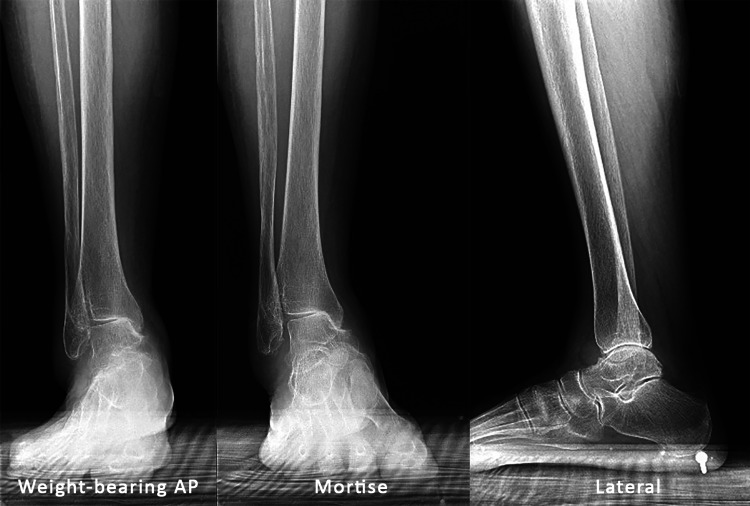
Right ankle: AP, mortise, and lateral radiographs AP, mortise, and lateral radiographs of the right ankle show tibiotalar osteoarthritis with varus hindfoot and talar tilt.
